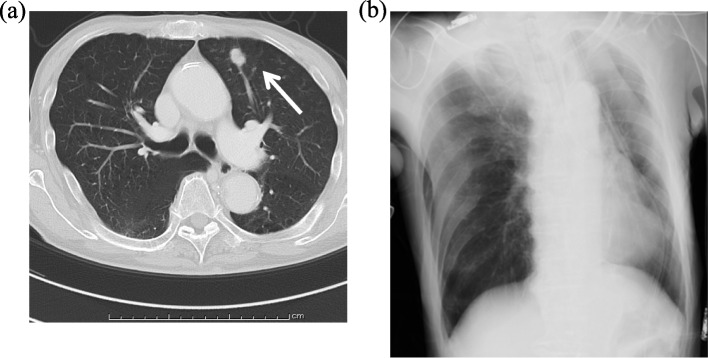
a Preoperative chest computed tomography. A preoperative chest computed tomography shows a 1.5-mm solid nodule with spicula in the left upper lobe (white arrow). b Chest radiograph taken soon after the surgery. A chest radiograph taken soon after the surgery shows that the drain tube is inserted in the direction of the pulmonary apex and that there is no worsening of cardiac enlargement
